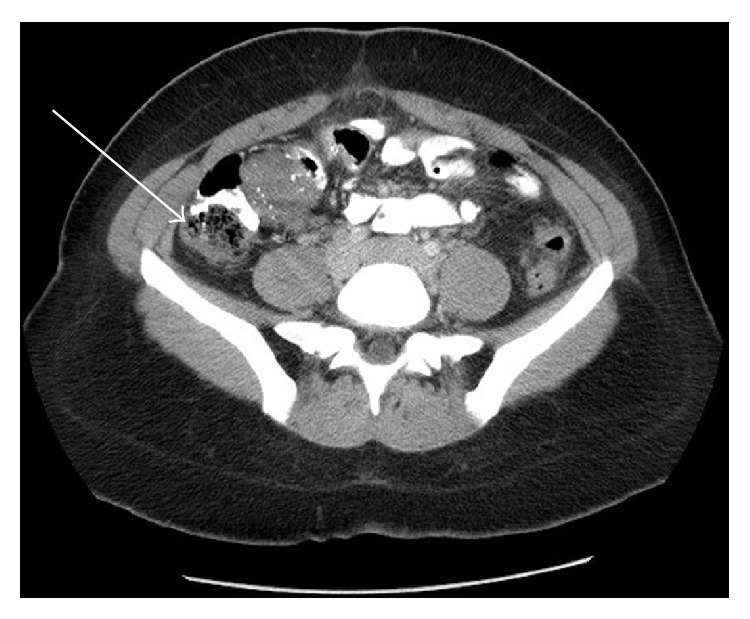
Computed tomography without contrast of the abdomen and pelvis was notable for a 4.7 cm mass involving the wall of the terminal ileum, with speckled calcifications, adjacent lymphadenopathy, and a small amount of dense fluid in the pelvis (CT, with arrow).
